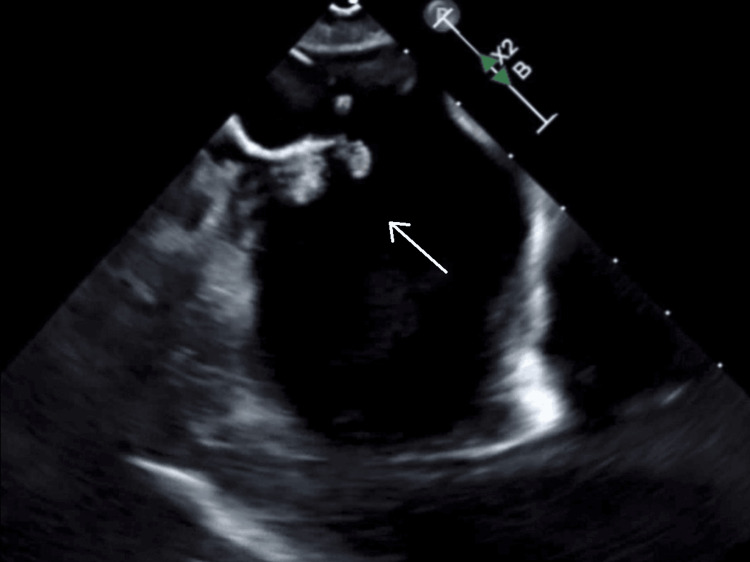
Transthoracic echocardiography (TEE) demonstrating an oscillating mass attached to Chiari network Transesophageal echocardiography demonstrating a prominent Chiari network with an attached oscillating echodense mass (white arrow), consistent with vegetation formation.
